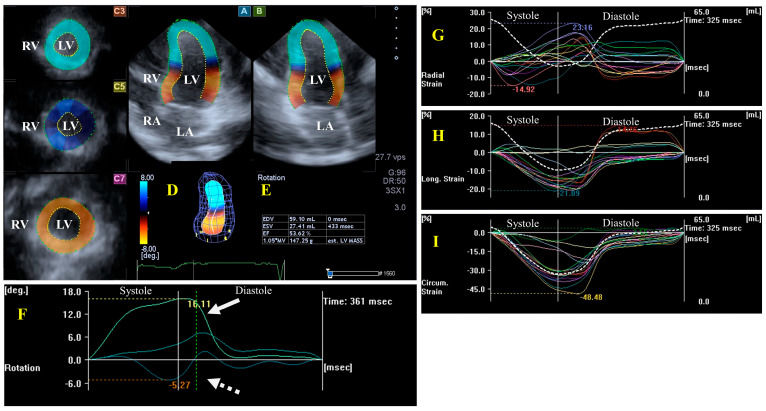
Evaluation of the left ventricle (LV) by three-dimensional (3D) speckle-tracking echocardiography. Typically, several views are automatically created during the LV assessment using a dedicated vendor-provided software: apical longitudinal four-chamber (A) and two-chamber (B) views and apical (C3), midventricular (C5), and basal (C7) short-axis views. In a 3D LV model (D), LV volumes, ejection fraction, and mass (E) and the apical (white arrow) and basal (white dashed arrow) rotations of the LV (F) are seen with time, as well as the LV global (white curve) and segmental (colored curves) radial (G), longitudinal (H) and circumferential (I) strain curves with time, and an LV volume changes curve with time (dashed white curve) are presented. Abbreviations: RA = right atrium; RV = right ventricle; MASS = LV muscle mass; LA = left atrium; LV = left ventricle; EF = ejection fraction; EDV = end-diastolic volume; and ESV = end-systolic volume.
